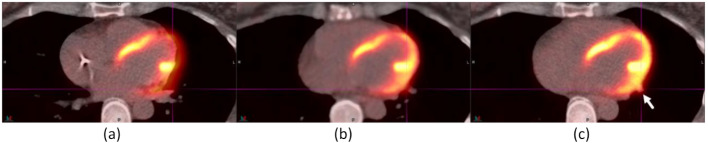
A 0.5-cm lung lesion (indicated by an arrow) was called in DDG CT (c) but not in baseline (a) or AIR (b). The SUV_max_ values of the lung nodule were 6.59 for baseline, 7.68 for AIR, and 7.96 for DDG CT
